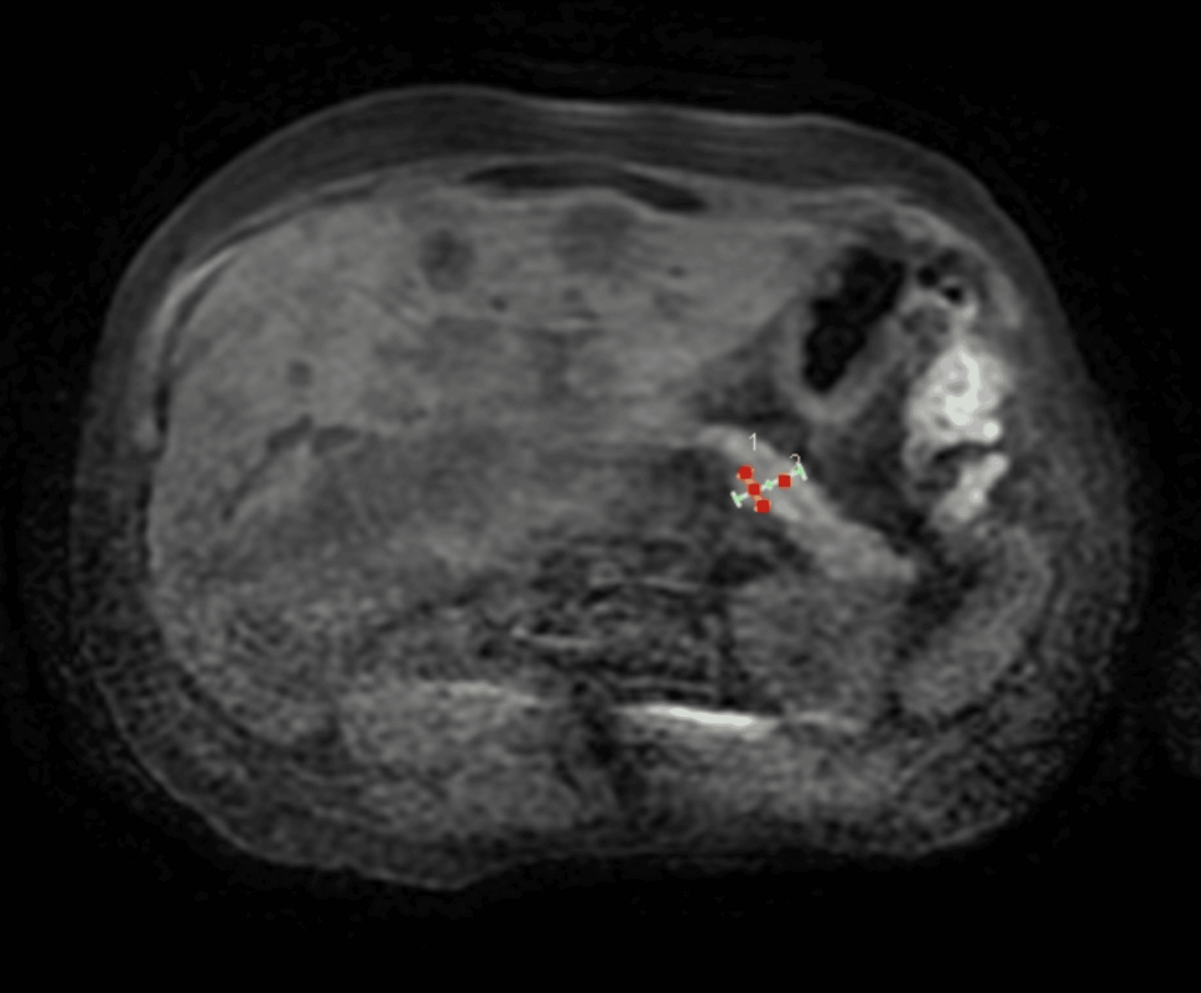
Abdominal and pelvic CT showing slight enhancement of the pancreatic duct at the junction of the body and tail.
